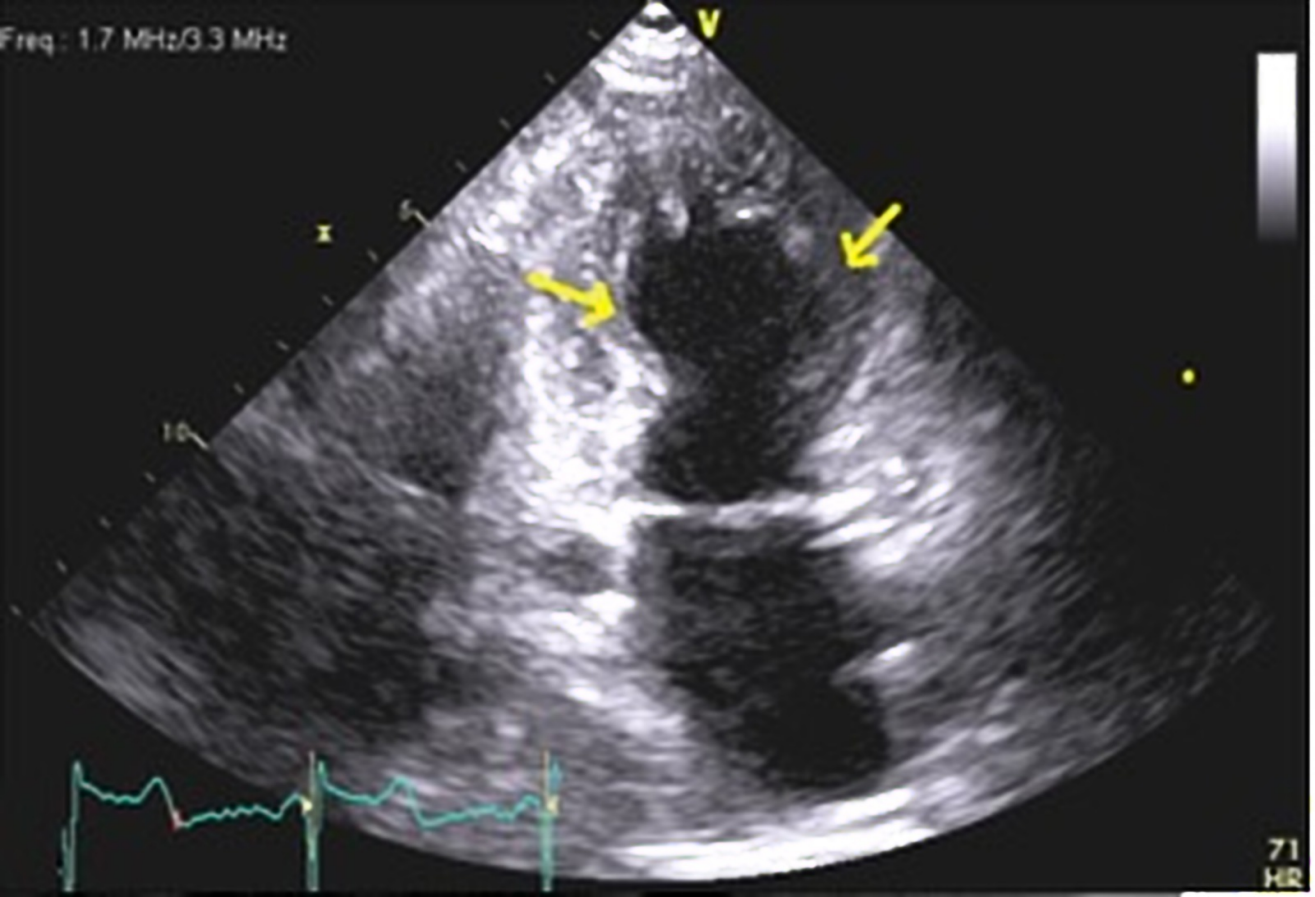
Transthoracic echocardiogram during systole, showing ballooning of the mid-left ventricular cavity
